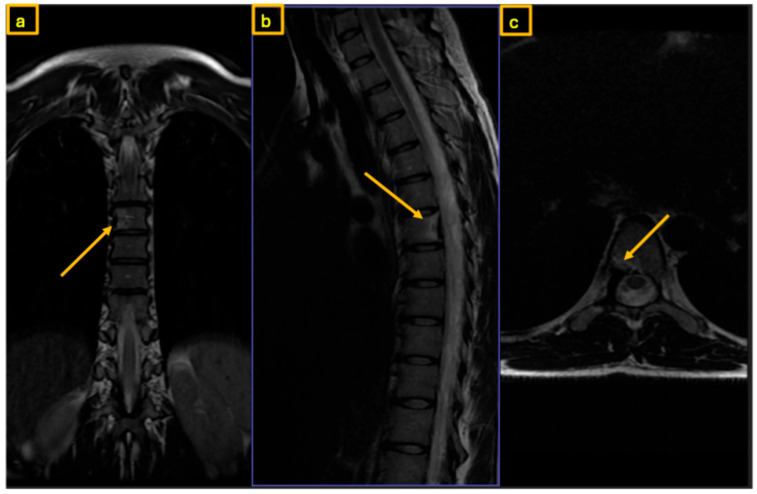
Preoperative MRI of the thoracic spine. The yellow arrow indicates the nidus at the root of the right T7 pedicle: (a) coronal view, (b) sagittal view, and (c) axial view.
